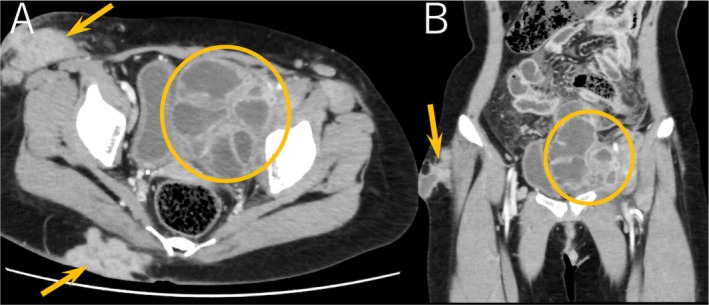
Contrast‐enhanced abdominal CT (A: axial view, B: coronal view) scan shows a 10.5 cm multilocular retroperitoneal abscess with rim enhancement extending from the pelvis to the left inguinal area (circle). Arrows indicate scars from cutaneous abscesses.
